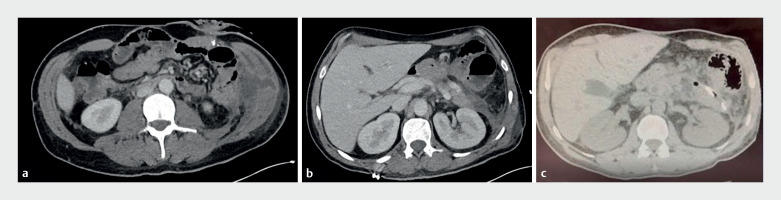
CT images before and after the procedure.
